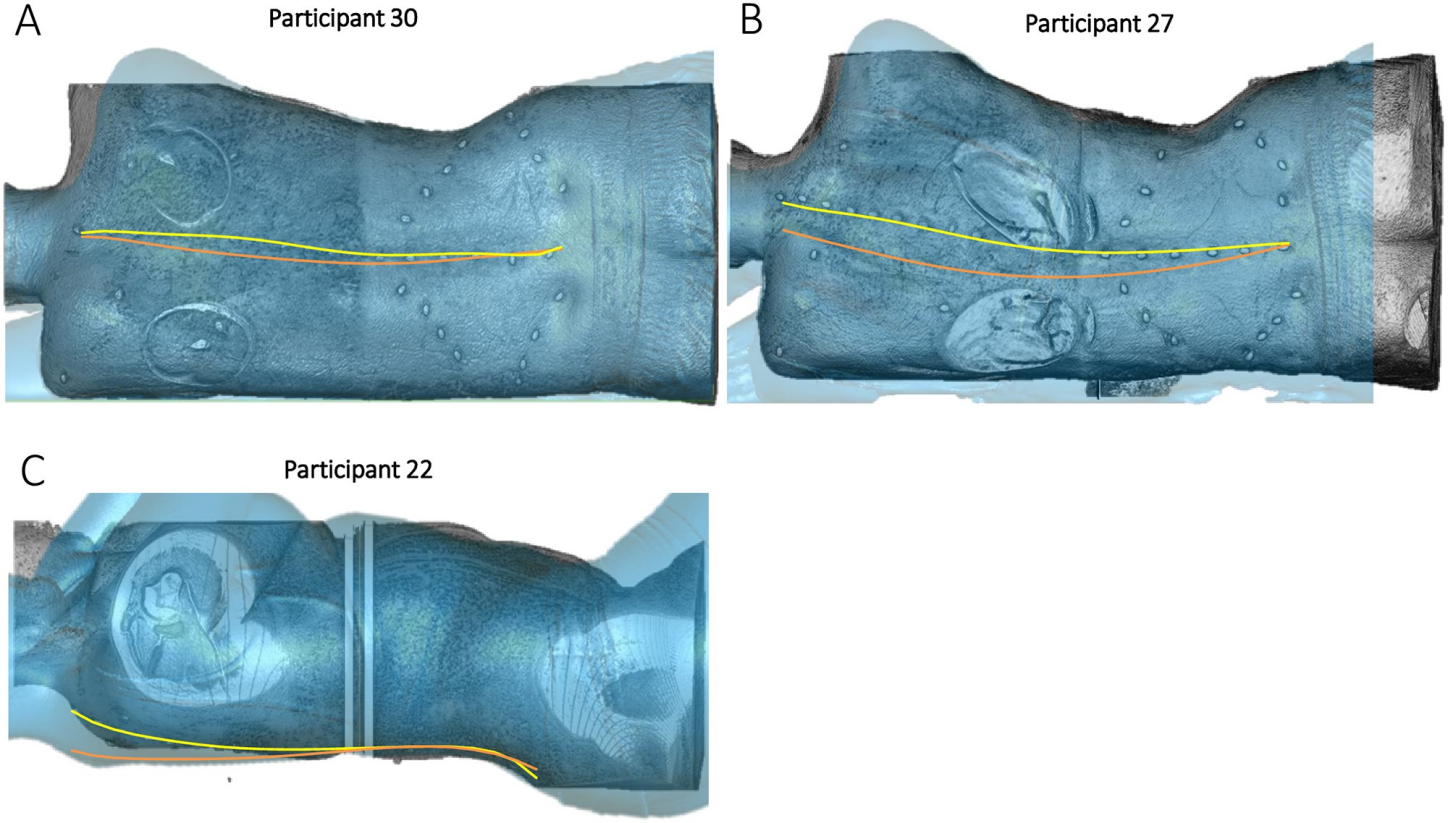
Comparison of the MRI 3D volume render and 3DSS. Comparison of FMP (yellow) and 3MP contours (orange) overlayed on a 3D volume rendering of the MR images (in Amira) shown in grayscale, with the 3DSS shown in transparent Blue (from Geomagic). In comparison to the 3DSS, participant 30 (A), shown in the coronal view, shows elevation of the right shoulder. Participant 27 (B), shown in the coronal view, shows more lumbar lateral flexion in the MRI. Participant 22 (C) shown in the sagittal view, demonstrates more lumbar lordosis and thoracic kyphosis.
